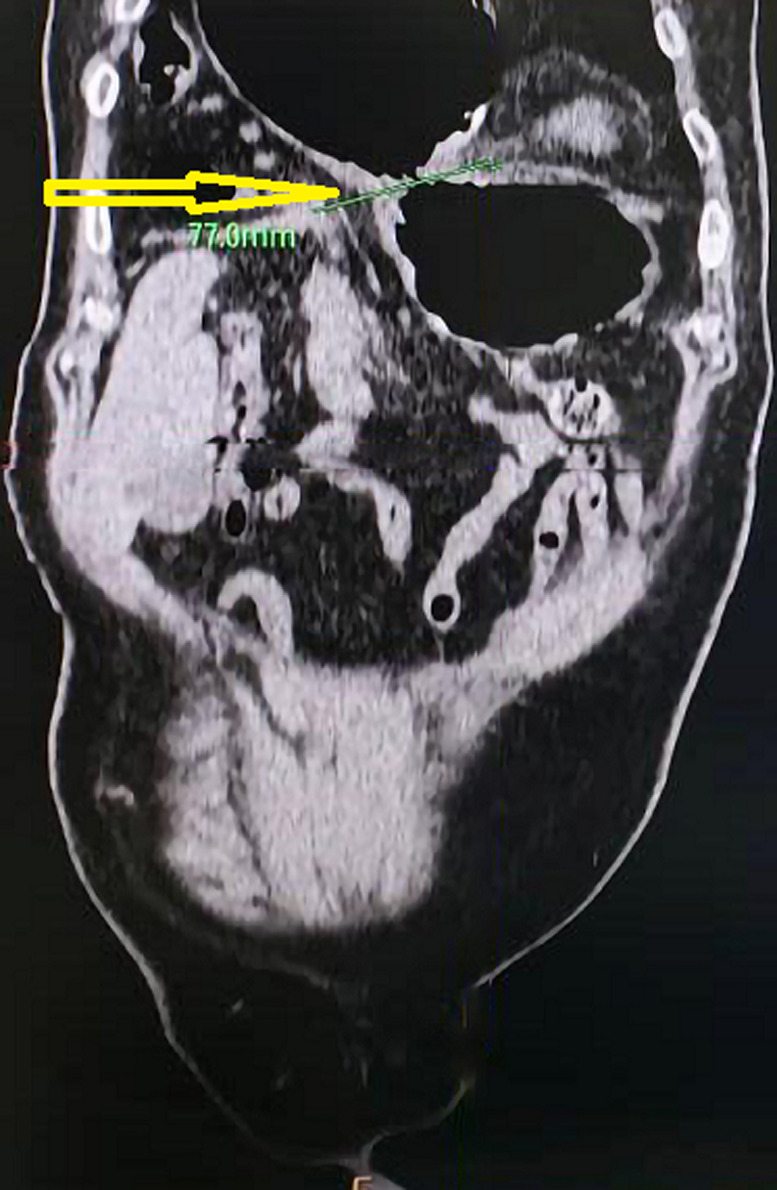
computed tomography scan showed the Morgagni hernia (arrow)
